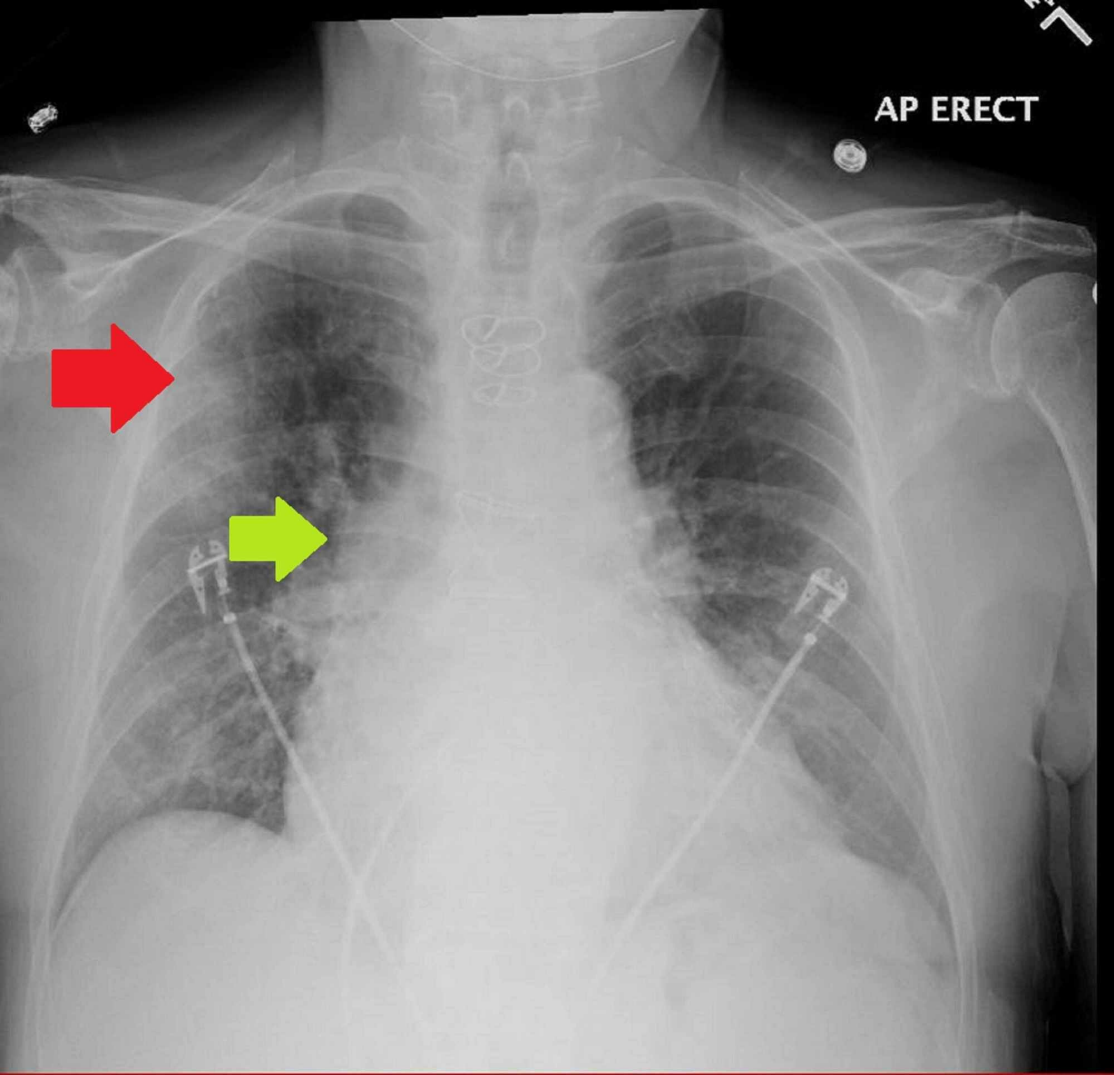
Chest X-ray on presentation showing hazy right upper lobe opacity (red arrow) with increased prominence of right hilum indicating adenopathy or inflammatory changes (green arrow)
